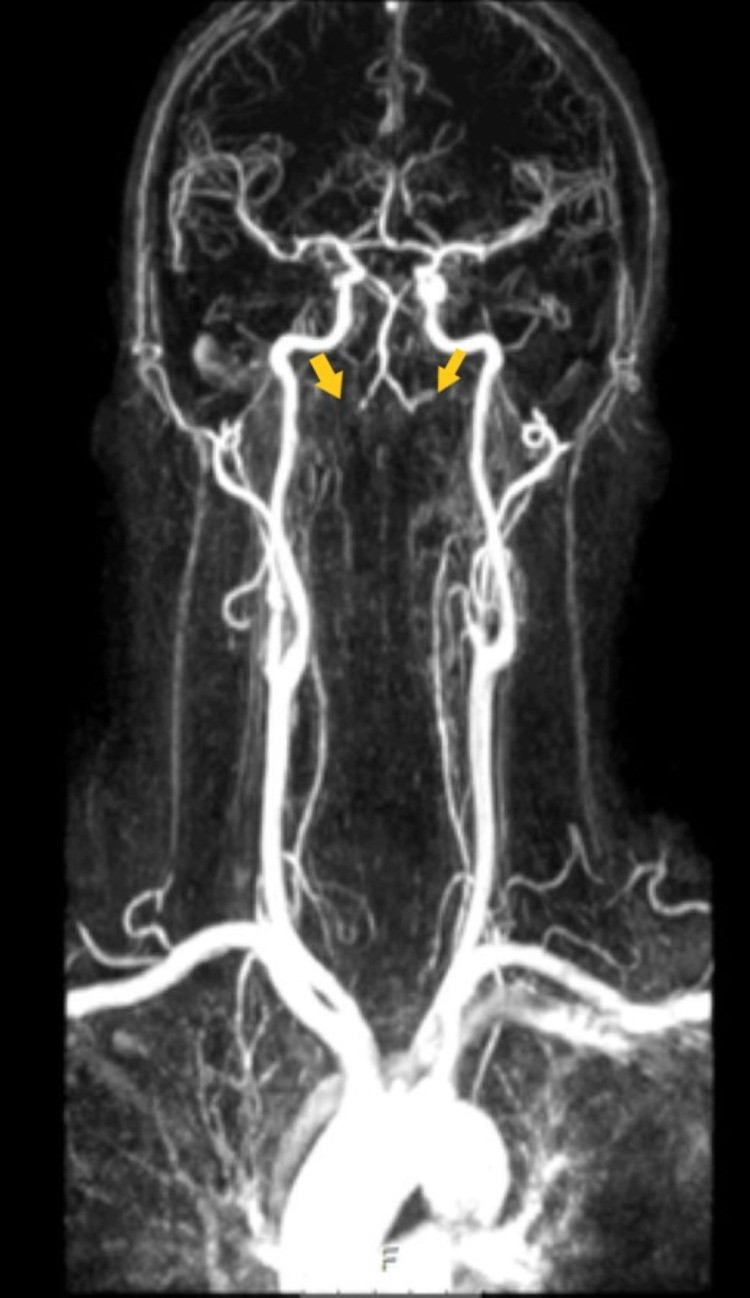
Brain angio-CT with bilateral vertebral occlusion. CT, computed tomography.
